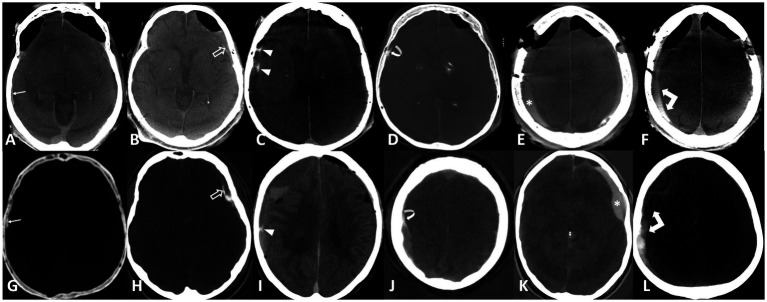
Enhancement patterns: Upper row flat-panel CT, lower row iodine maps of photon counting CT. (A,G) Outer membrane (arrows), (B,H) inner membrane (open arrows), (C,I) septations (arrowheads), (D,J) spandrel sign (curved arrows), (E,K) internal enhancement (asterisk), (F,L) fluid–fluid level (double arrows).
